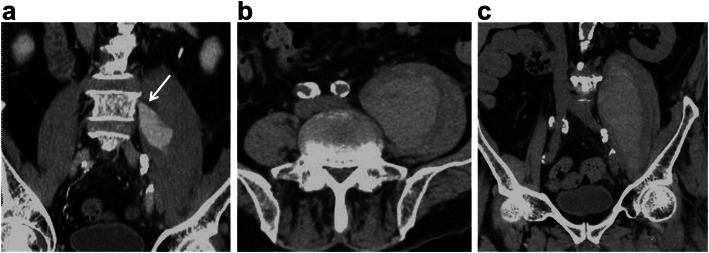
Computed tomography of developing giant psoas muscle hematoma. a Computed tomography angiography on day 8 after admission shows the contrast medium leakage (arrow) indicating continuation of bleeding from the vertebral segmental artery. b,c Tense swellings of the left psoas major muscle are shown in plain computed tomography on day 14 after admission
